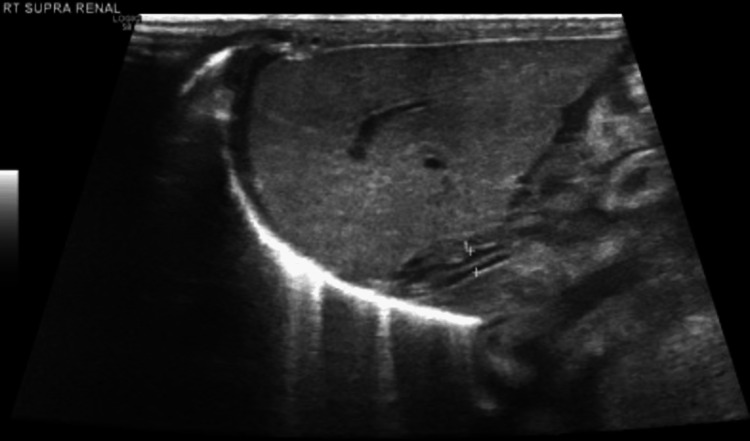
Abdominal ultrasound (20 minutes later) showing the size of right suprarenal gland decreased to normal.
